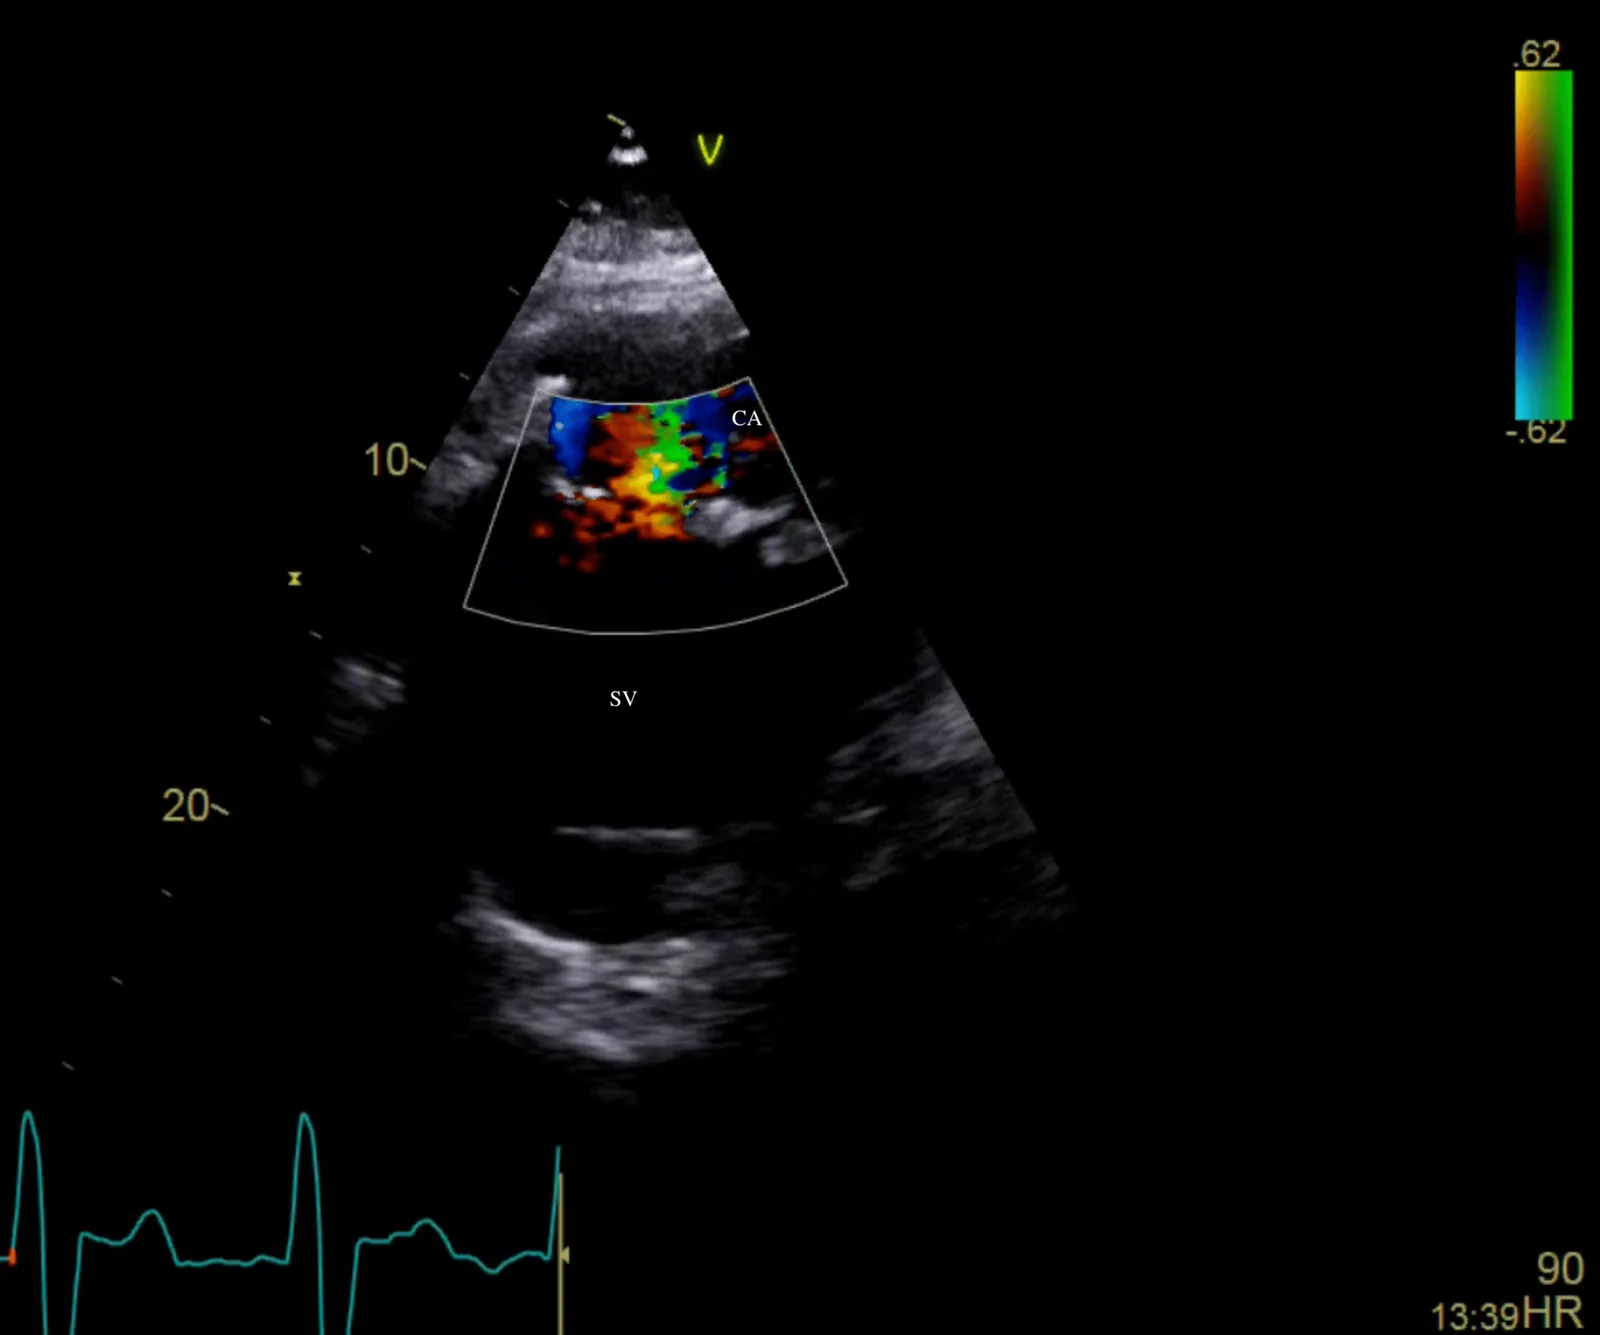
Transthoracic echocardiographic image demonstrating single-ventricle anatomy and severe common AV valve regurgitation SV: single ventricular chamber, CA: common atrium, AV: atrioventricular
